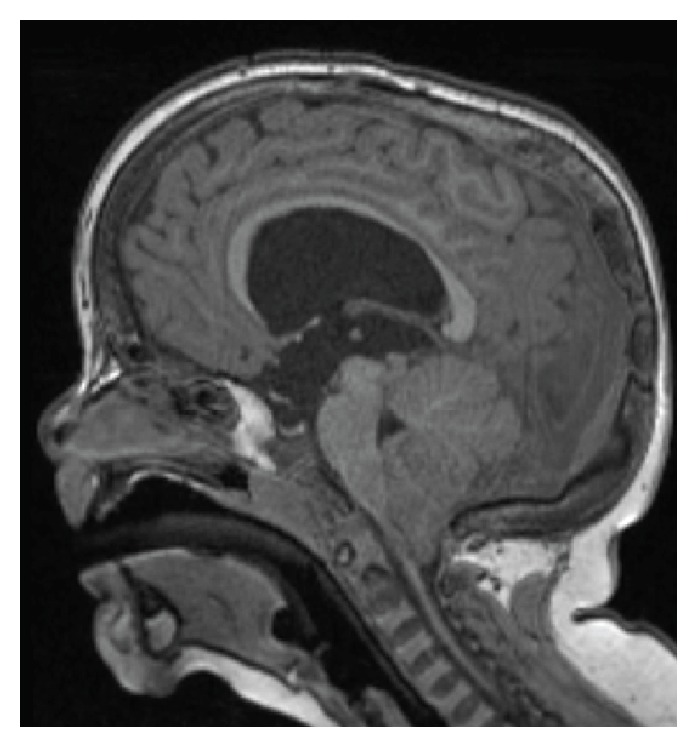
Brain MRI showing hydrocephalus and CM-I.
